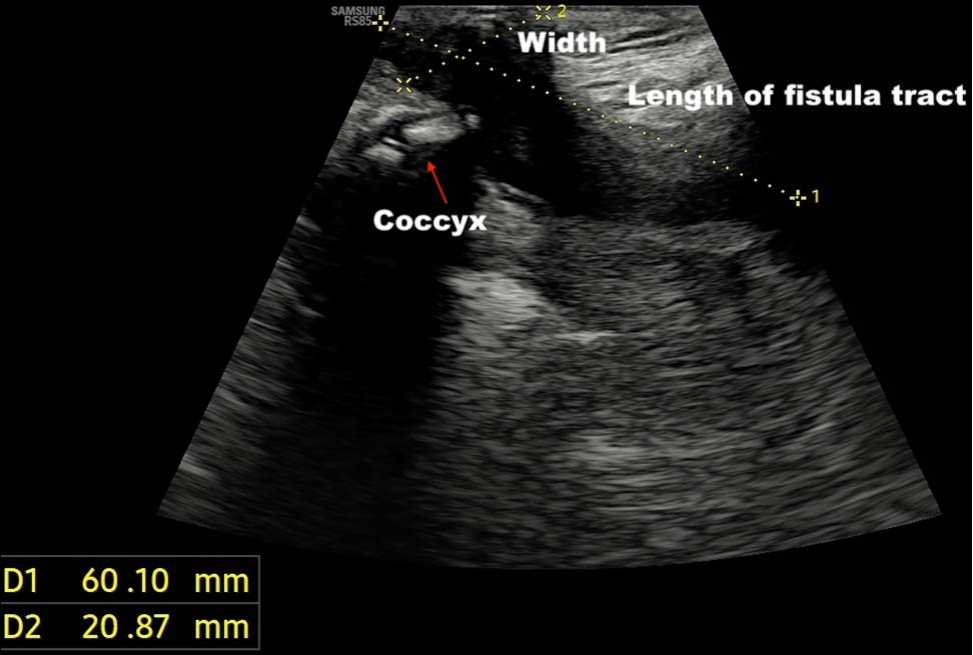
Transperineal ultrasound image revealing posterior extension of the fistulous tract reaching up to the level of the coccyx.
